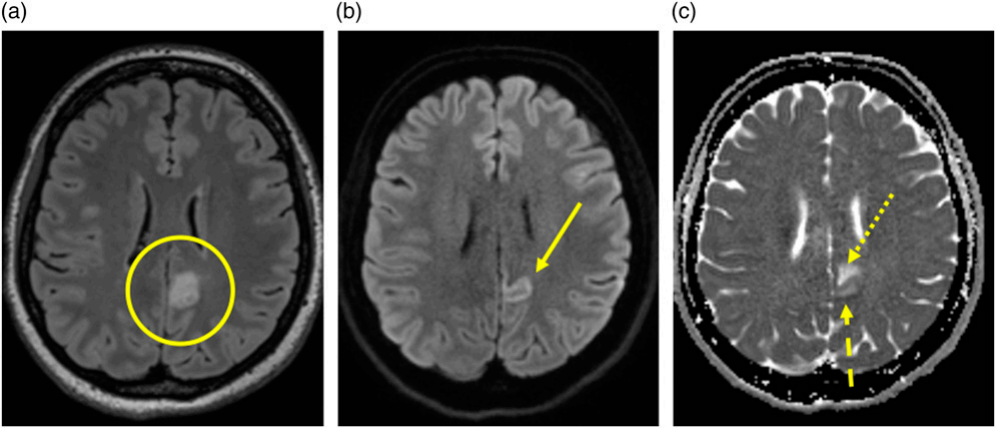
The split ADC sign in autoimmune encephalitis. (a) FLAIR image demonstrating signal change within the cortex and subcortical white matter of the left posterior cingulate gyrus (circle). (b) DWI image demonstrating signal change on DWI within the cortex (arrow). (c) ADC map demonstrating low ADC within the cortex (dashed arrow) and high ADC within the subcortical white matter (dotted arrow).
